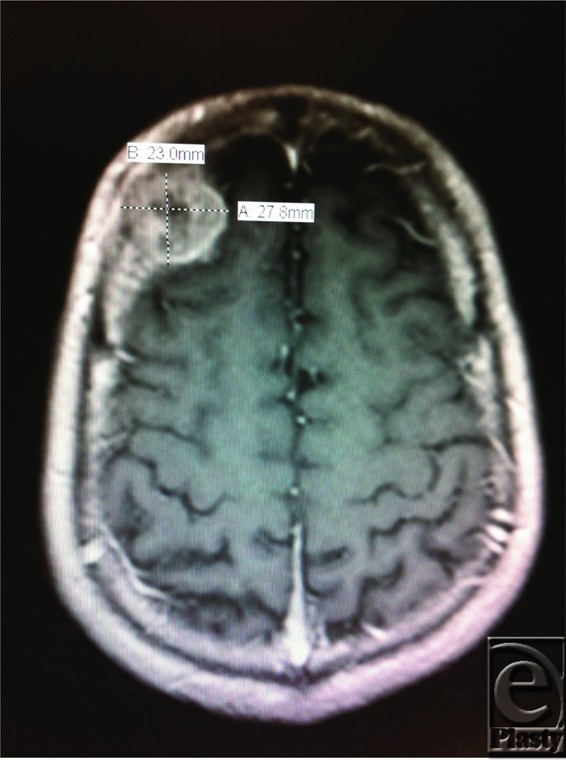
Right image: MRI of the head axial view showing 2.3 × 2.8 cm^2^ intracranial mass. Images taken following subcutaneous incisional biopsy.
